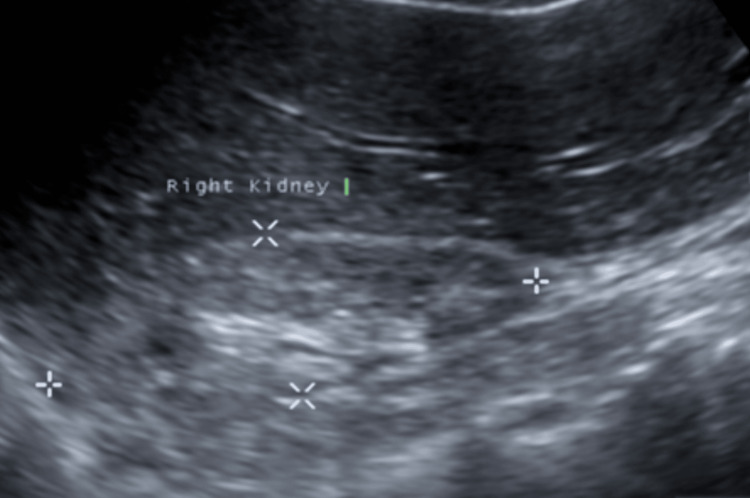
Retroperitoneal ultrasound showing an atrophied right kidney.
